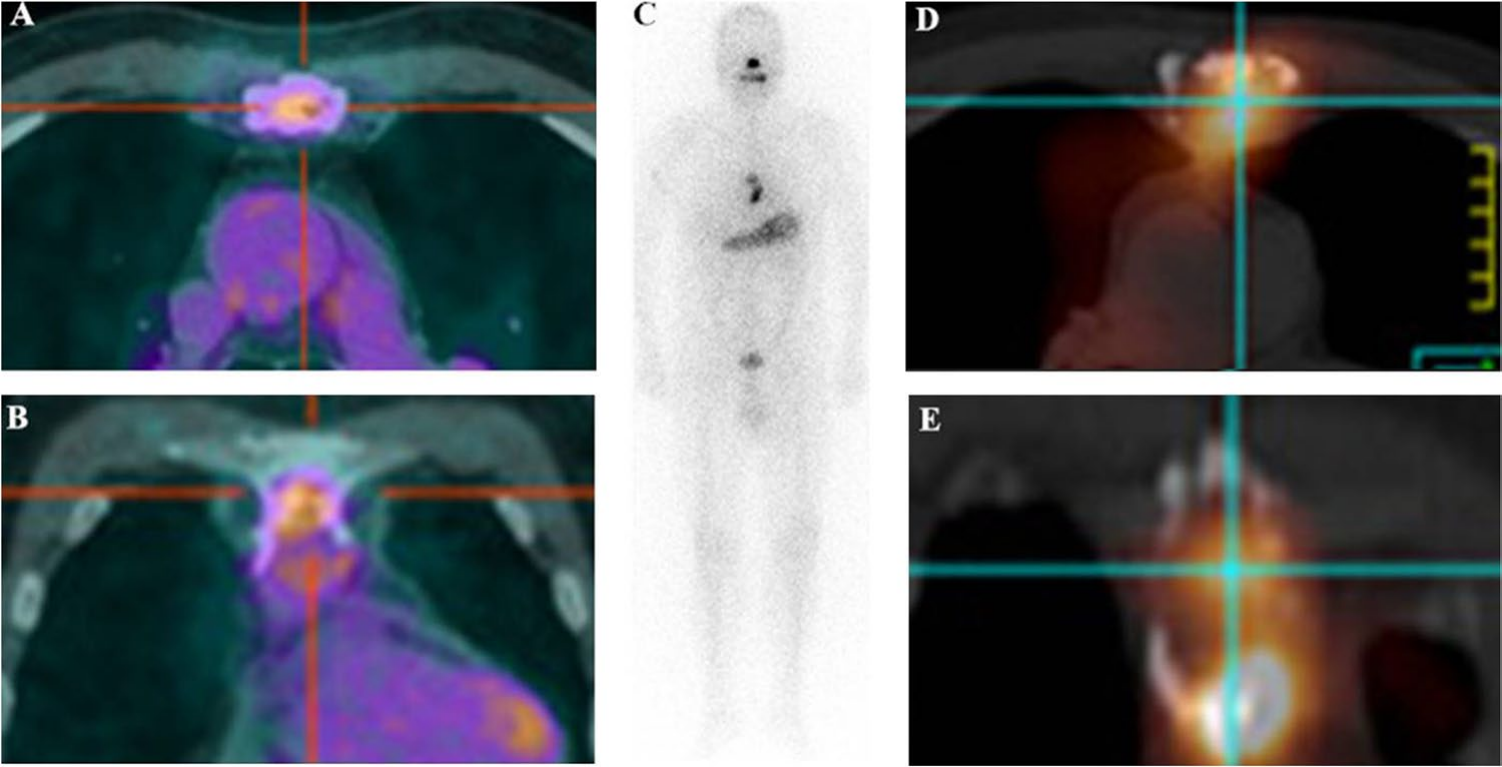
^18^F-FDG-avid papillary thyroid carcinoma (PTC) metastatic sternum lesions avid for ^131^I. A 55-y-old male patient with PTC presented with suspected metastatic disease one month after total thyroidectomy with ssTg of 604.7 ng/mL and TSH of 60.2 mU/L. Transaxial (A) and coronal (B) image of ^18^F-FDG PET/CT before ^131^I therapy showed sternum lesions with increased ^18^F-FDG uptake (SUVmax = 3.6). ^131^I planar image (C) and SPECT/CT (D,F) after the initial administration of 7.4 MBq (200 mCi) ^131^I correspondingly showed the sternum metastases with increased ^131^I accumulation. Six months later, ssTg decreased to 125.9 ng/mL under TSH stimulation by levothyroxin withdrawl just before the second course of ^131^I administration.
